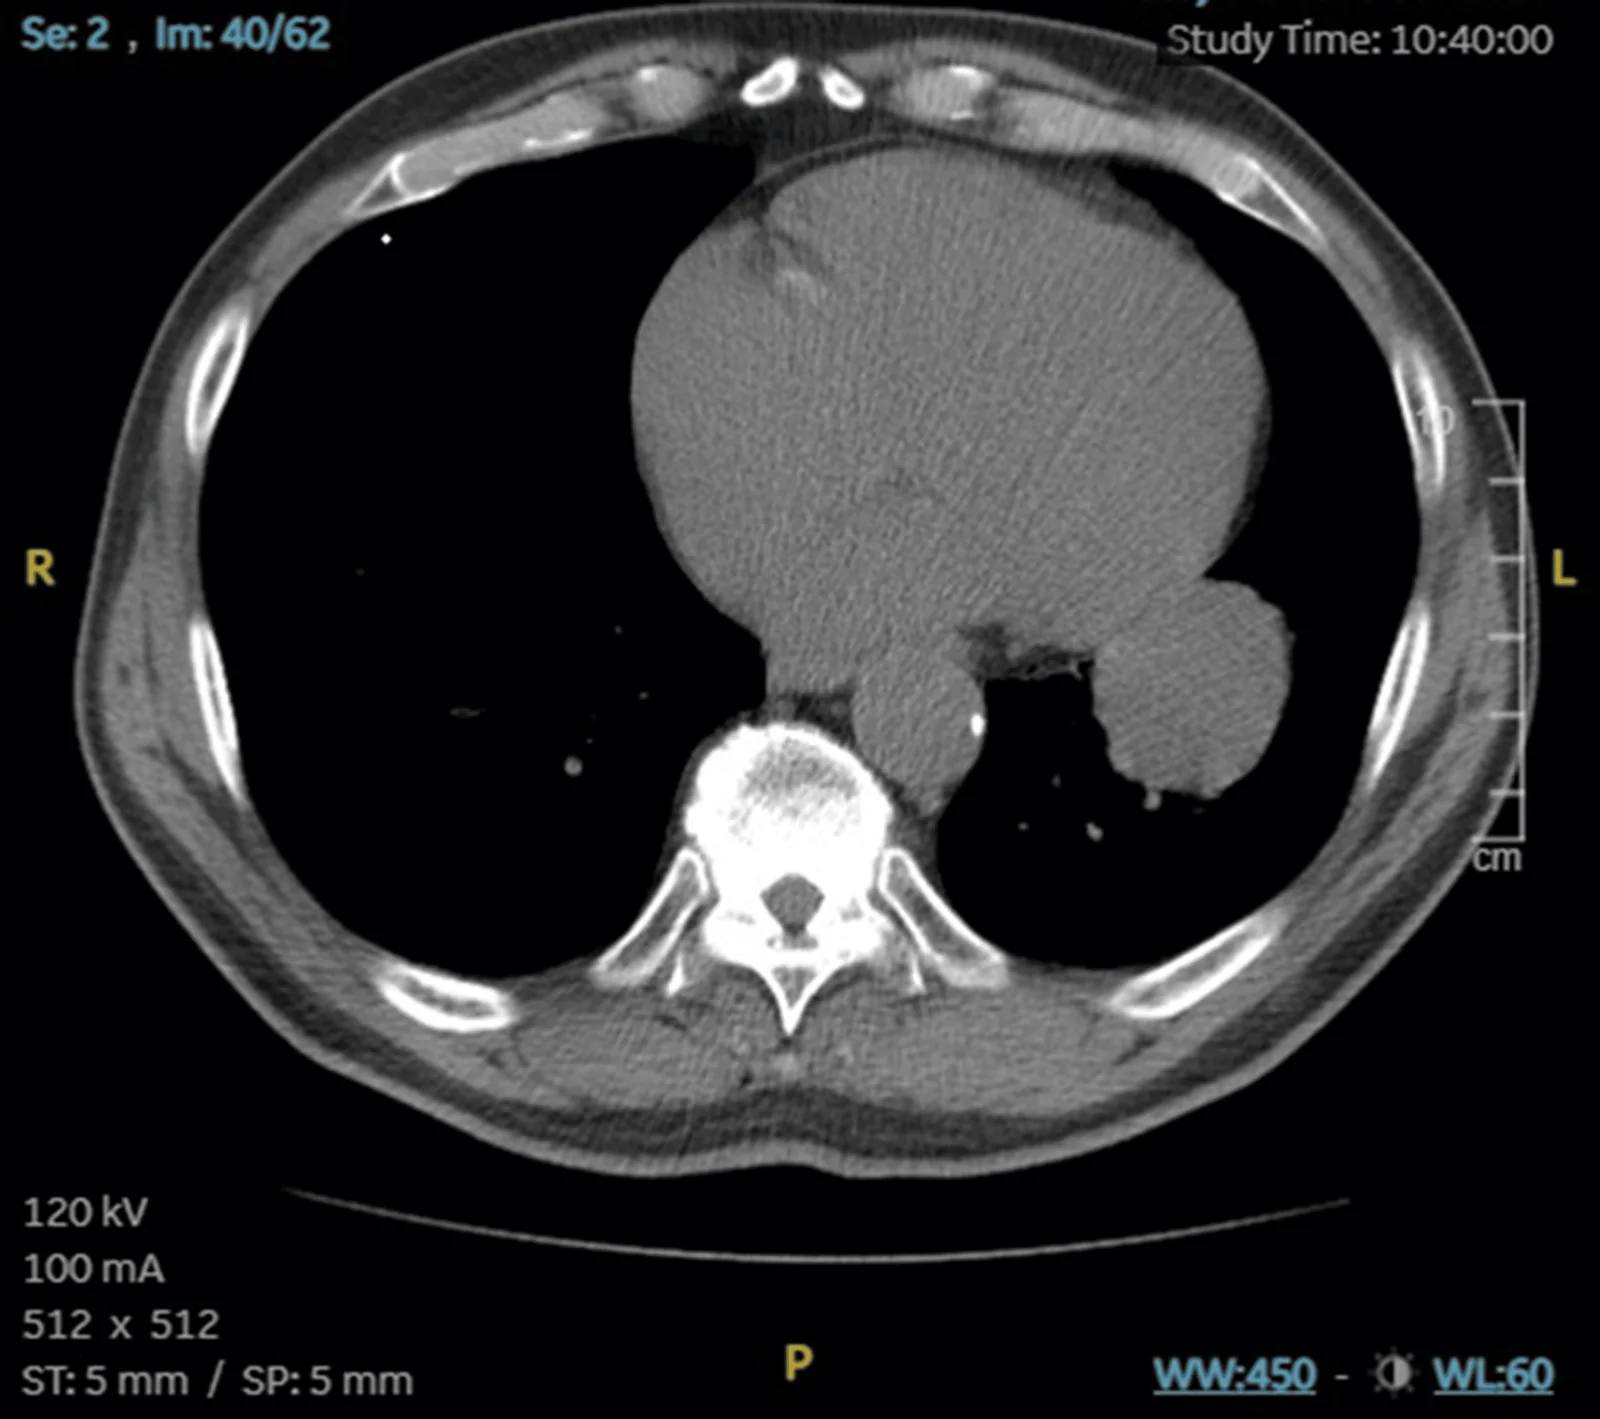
Non-contrast CT chest demonstrating a new left lower lobe pulmonary mass with soft-tissue attenuation Axial non-contrast CT of the chest demonstrates a newly identified left lower lobe pulmonary mass measuring 4.5 × 5.3 × 5.0 cm. The lesion demonstrates soft-tissue attenuation with an average density of approximately 36 HU on soft-tissue window imaging CT: computed tomography
